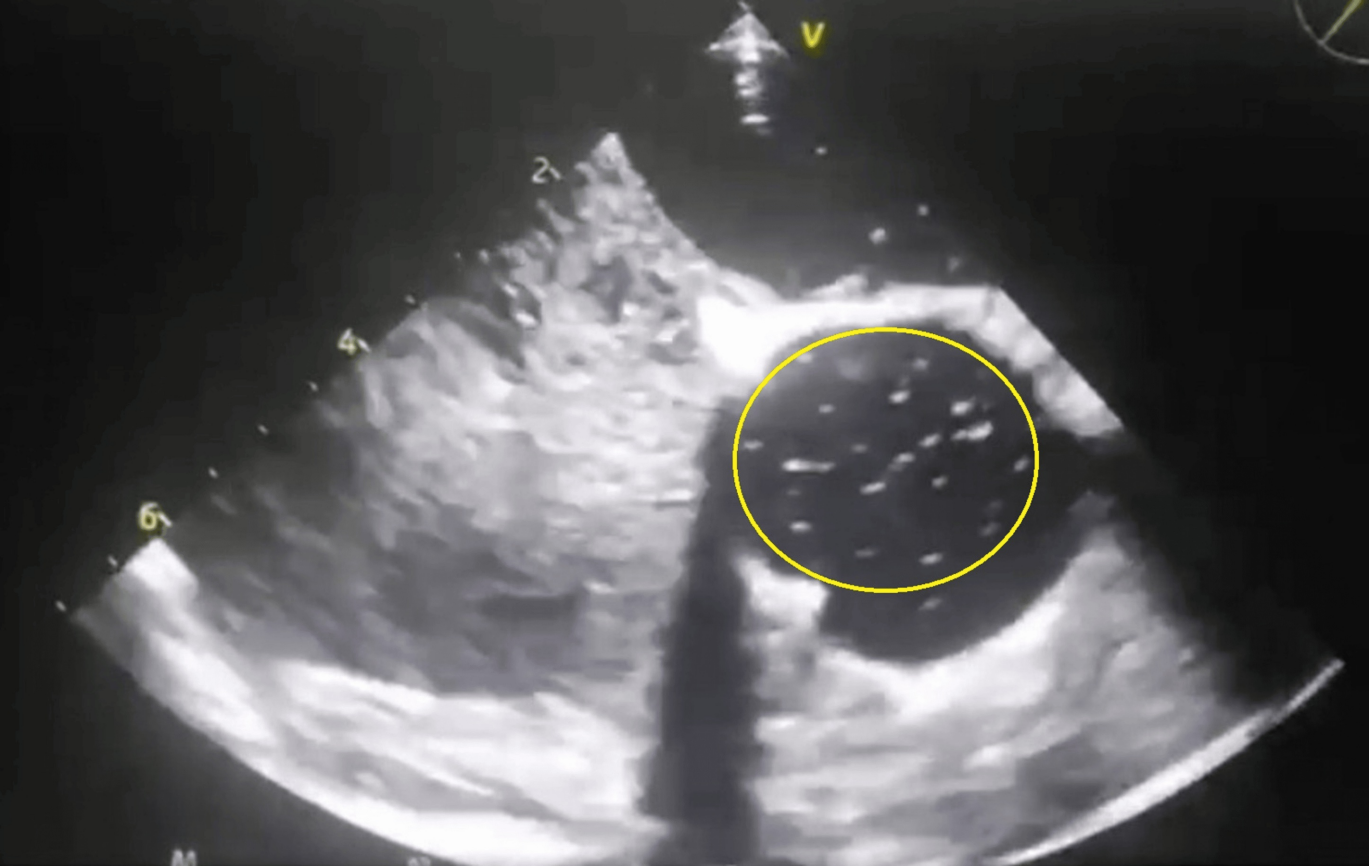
Bubble-contrast transesophageal echocardiogram (TEE) confirming the presence of a patent foramen ovale (PFO). More than 20 microbubbles are seen crossing from the right atrium to the left atrium within three cardiac cycles, consistent with a large right-to-left shunt. The study was performed with a Valsalva maneuver to accentuate shunting. No precise measurements of tunnel length or septal mobility were obtained, which is acknowledged as a limitation. The area of shunting is highlighted with a yellow circle.
